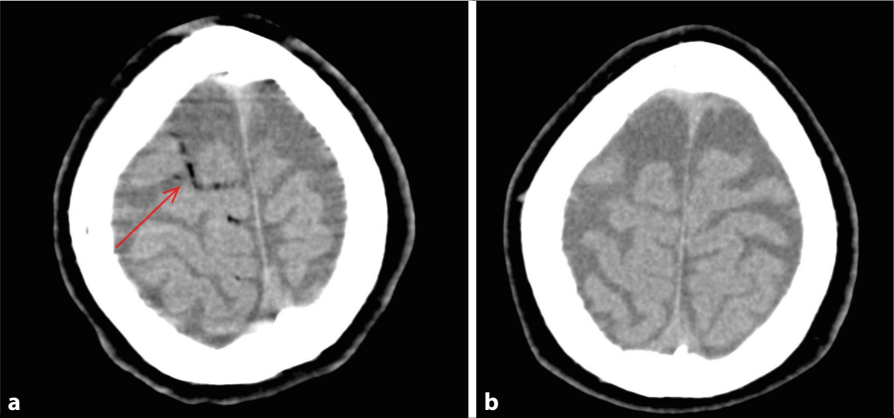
a Axiale kraniale CT-Aufnahme unmittelbar nach dem Ereignis. Darstellung von hypodensen, luftäquivalenten Strukturen in den oberflächlichen kortikalen Hirnvenen (siehe *roter Pfeil*), vereinbar mit einer zerebralen Luftembolie. b Kontroll-CT nach 17 h. Vollständige Regression der zuvor nachgewiesenen intrazerebralen Lufteinschlüsse, korrelierend mit der klinischen Besserung
